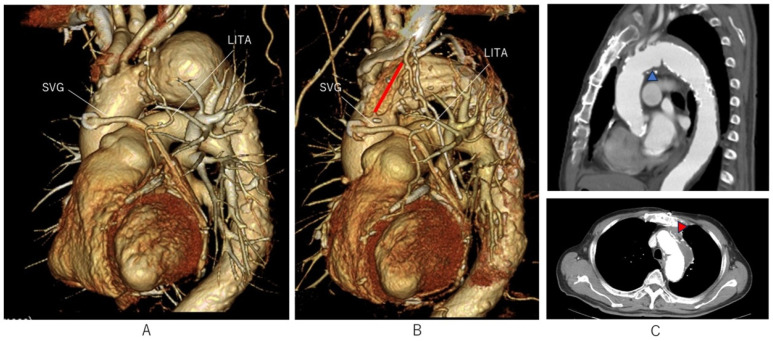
Example of a distal arch aneurysm with patent bypass grafts. (A) Preoperative three-dimensional CT reconstruction demonstrating patent LITA and SVG grafts. (B) Postoperative reconstruction showing preservation of the ascending aorta and proximal fixation at the aortotomy closure site (red line). (C) Postoperative CT images confirming secure incorporation of the proximal graft within the native arch. The sagittal view (upper panel) demonstrates the proximal fixation site (blue arrowhead), and the axial view (lower panel) shows the sutured closure line reinforced with felt (red arrowhead).
